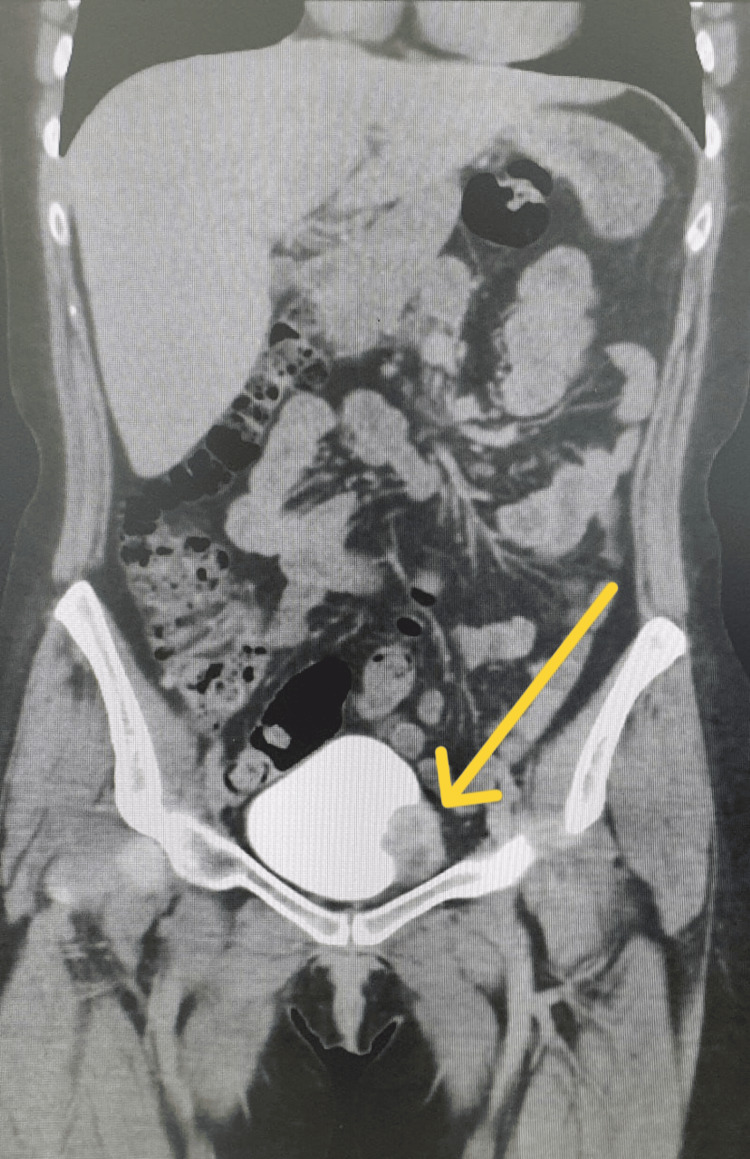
Computed tomography in coronal view showing left lateral wall bladder lesion 3 cm × 2 cm.
